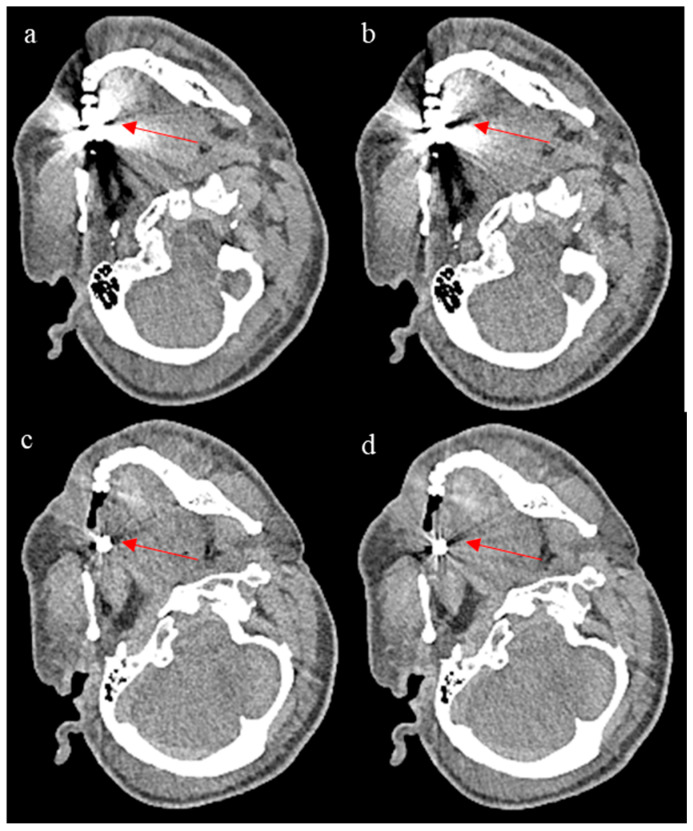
The figure shows ErisNet’s performance on CT images containing metallic artefacts. (a,c) are images processed with ErisNet showing noise reduction compared to the corresponding low-dose inputs (b,d), respectively. The red arrows highlight specific regions where noise reduction in metal artefacts can be appreciated in the processed images. Although both cases (a,c) show some degree of noise reduction, as indicated by the arrows, the extent and quality of the improvement vary between the two scenarios.
